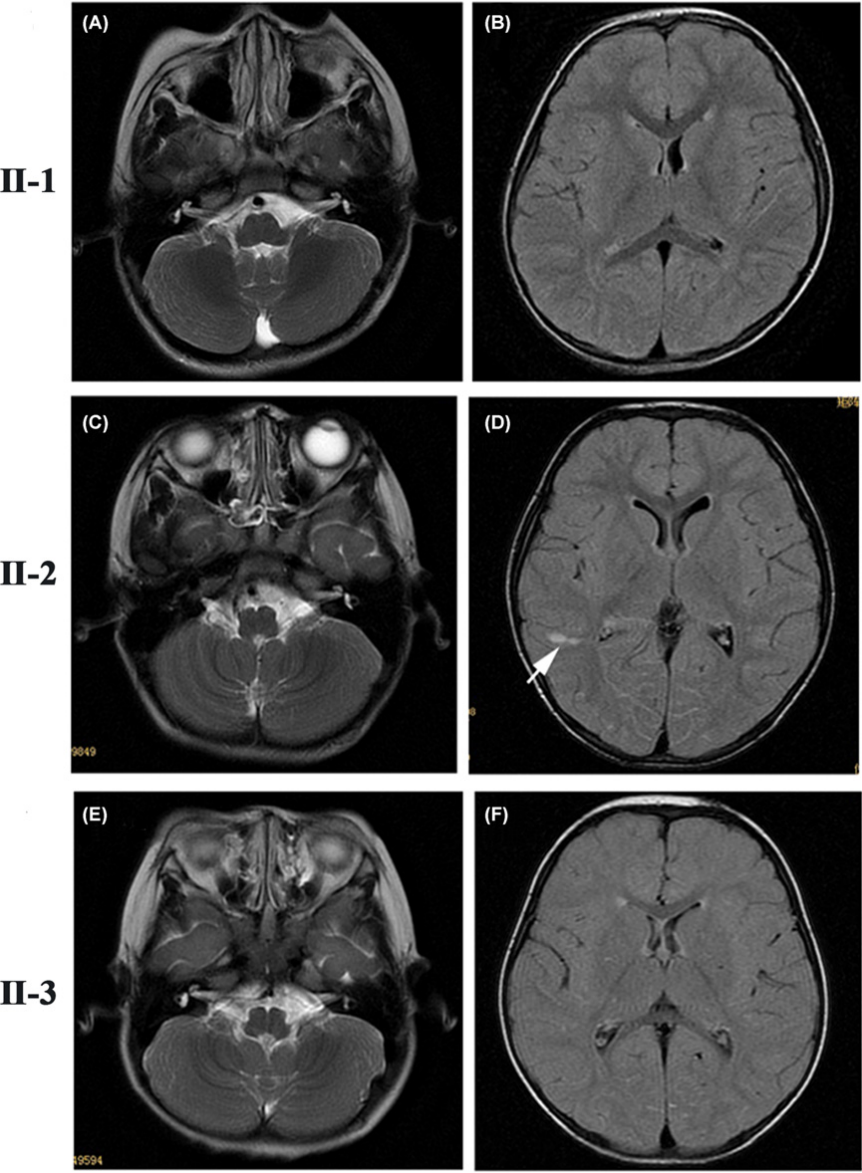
Results of MRI indicated no recognizable deficiency in the brain of the three patients of studied WS2 family The MRI images of the children (II-3, II-2 and II-1) showed some minor variations but no recognizable deficiency. (A,B) The MRI image of II-3 indicated that the white matter signal in the posterior angle of the bilateral ventricle and the half-oval center was altered. Clinical diagnosis suggested that it should consider as the end band. (C,D) The MRI result of II-2 indicated the presence of several abnormal signals such as point and flake shapes in right temporal lobe and left parietal lobe. Small-type circular cystic lesions appeared in the left hippocampus, which may suggest choroidal plexus cyst. In addition, thickening of the bilateral maxillary sinus, ethmoid sinus and sphenoid sinus mucosa was observed. (E,F) II-1 showed no abnormality.
